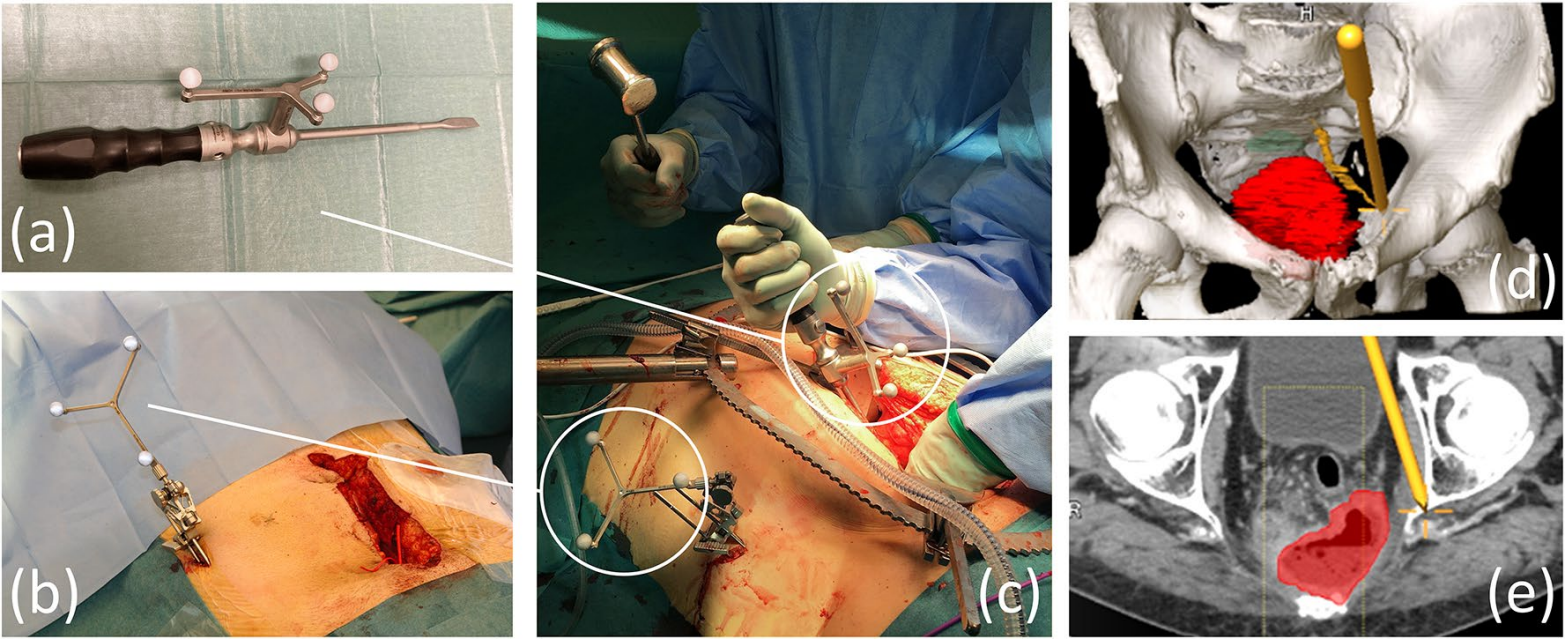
Intraoperative navigation. a Reflective spheres on chisel. b Reflective spheres on patient tracker. c Resection of the sciatic spine using a navigated chisel. d The chisel visualised in the 3D model. e The chisel visualised in the axial CT image containing the tip of the chisel
